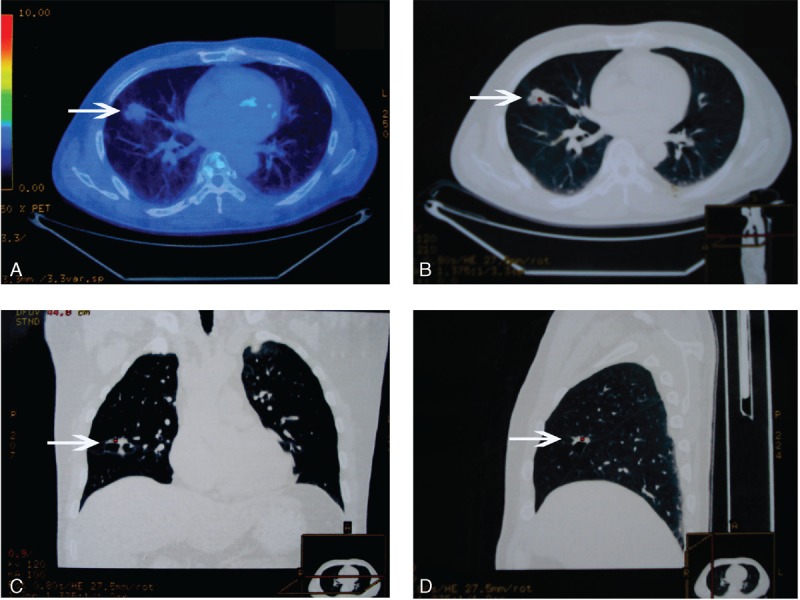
The ^18^F-FDG-PET/CT imaging of the lung lesion. (A) is the fused PET/CT image. (B), (C), and (D) are the CT images which were cross sections, coronal plane, and vertical plane based on the tumor, respectively. The lesion was pointed by white arrows.
